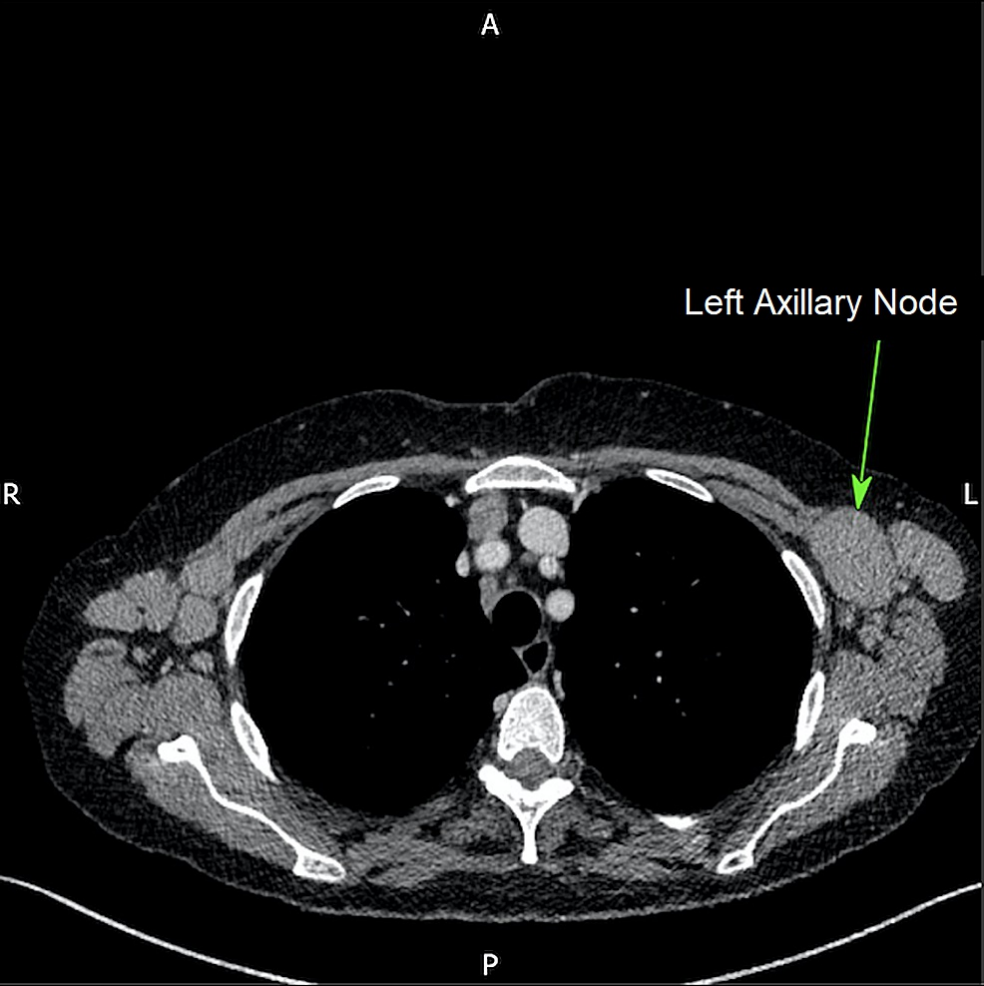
Computed tomography (CT) of the thorax displaying enlarged left axillary lymph nodes (green arrow).
